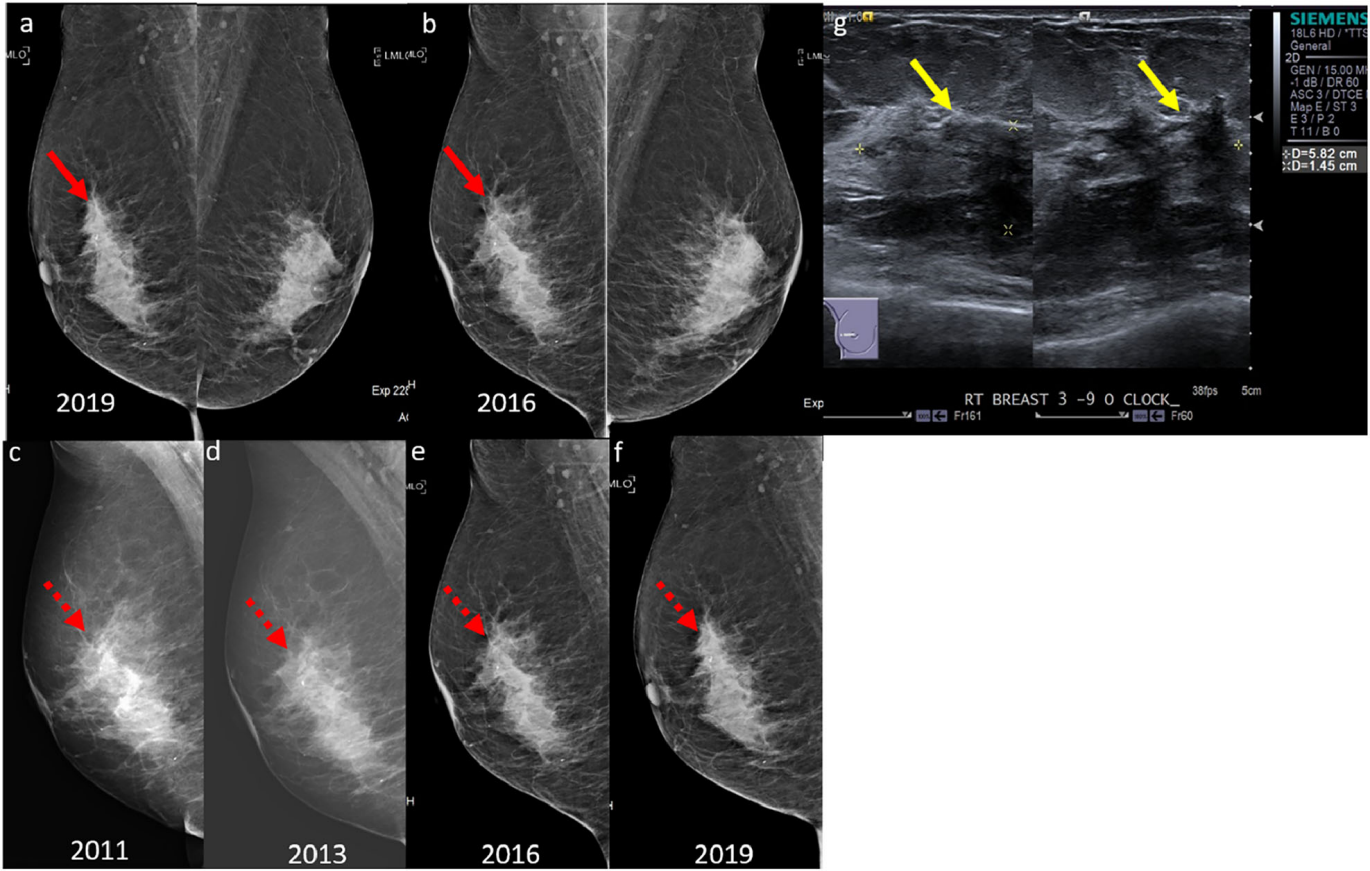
a A 49-year-old woman presented for an annual screening mammogram in 2019. Bilateral MLO mammograms reveal dense fibroglandular tissue with possible architectural distortion in the upper right breast (red arrow). b Comparison with prior mammograms dating back to 2016 showed stability of this finding. c–f Further comparison with multiple prior mammograms dating back to 2011 revealed progressive, but subtle increased conspicuity (dotted red arrows) of the architectural distortion. g The patient was recalled for a targeted ultrasound that revealed an irregular, hypoechoic non-mass lesion (yellow arrows) with mixed posterior features. Ultrasound-guided biopsy revealed grade 1 invasive lobular carcinoma. The case highlights the insidious and slow-growing nature of invasive lobular carcinoma, which can mimic stability over short intervals and be challenging to detect on mammography, especially in dense breasts
